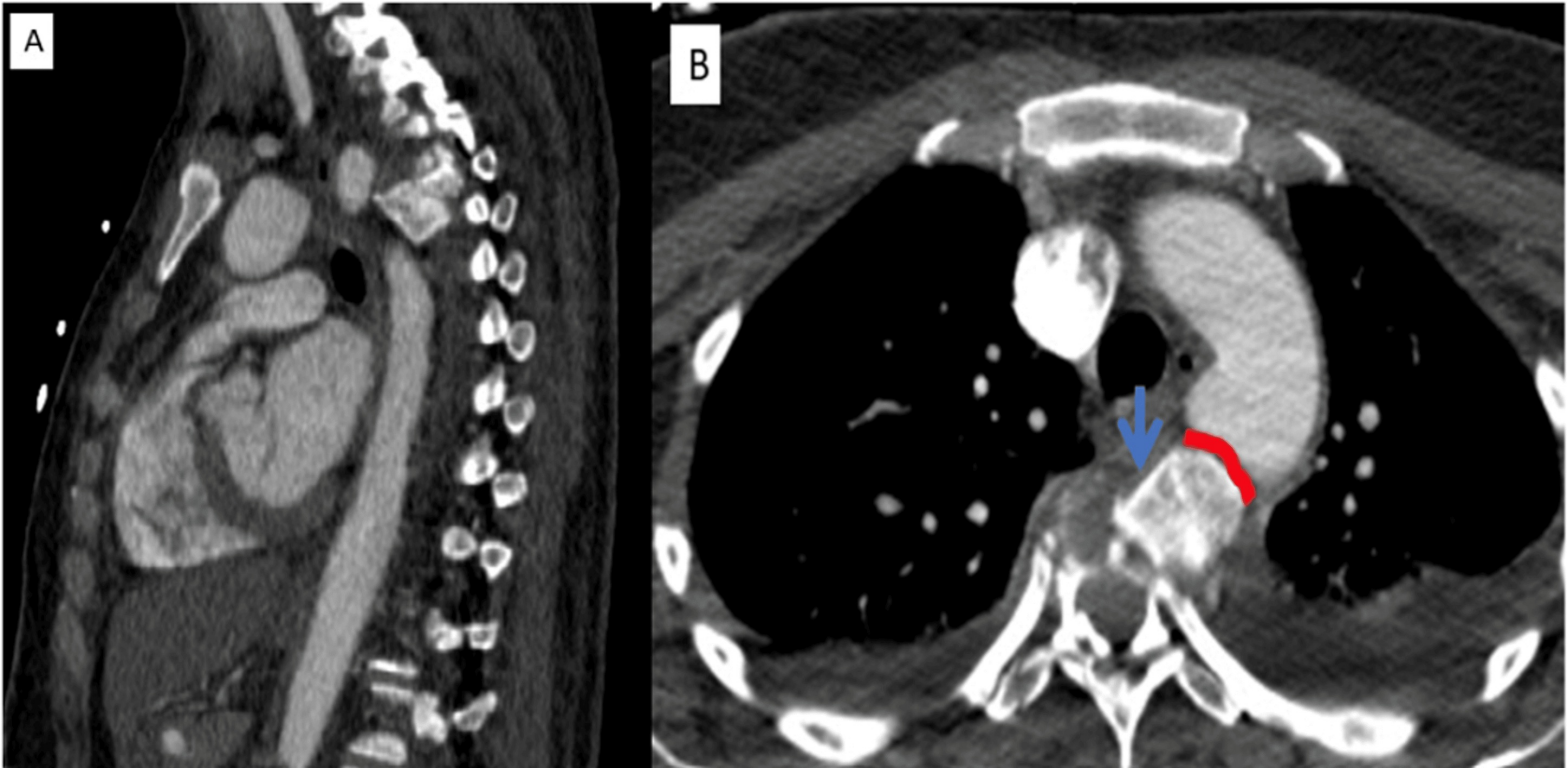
Sagittal (A) and axial (B) CT angiography Compression fracture with left anterolateral displacement of the fourth dorsal vertebrae (blue arrow), encroaching the posterior side of the aortic arch and the origin of the right subclavian artery, with a maintained rim of separation (red line)
